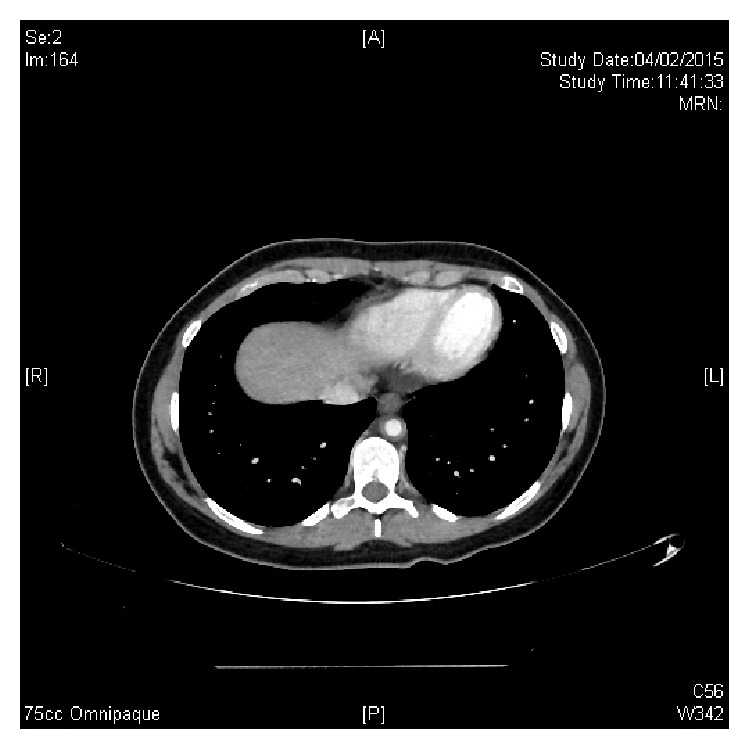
CT angiography revealing circumferential mural thickening of the lower part of the thoracic aorta.
